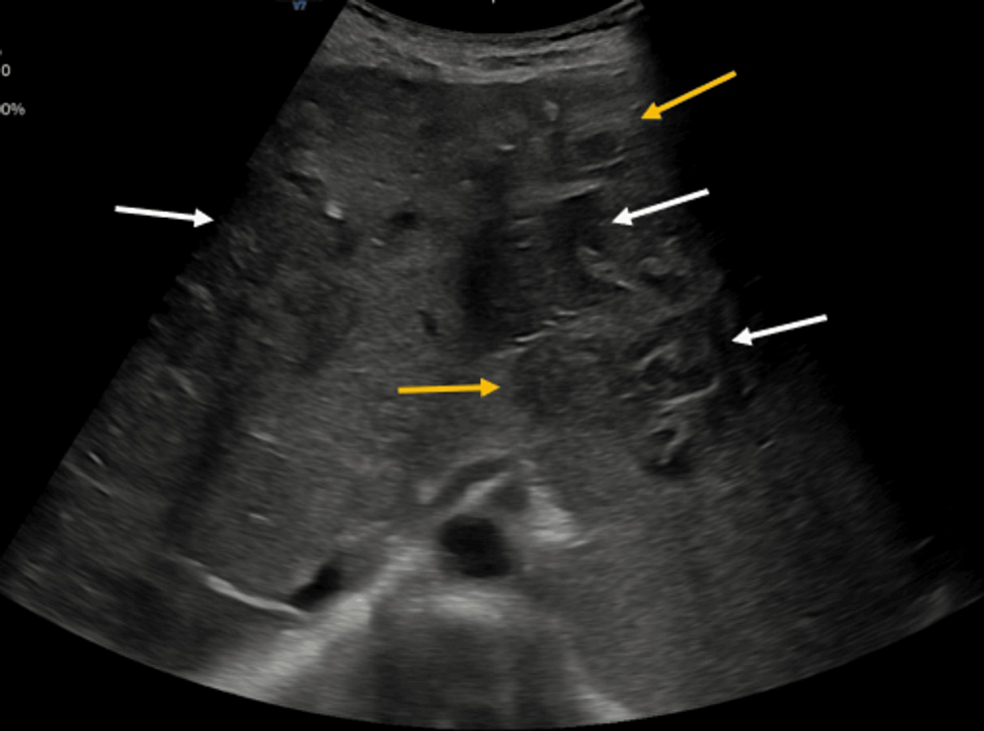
On ultrasound, multiple hypoechoic lesions are noted scattered throughout the liver parenchyma (white arrows). Some lesions show a target ring appearance that is a central hypoechoic area surrounded by hyperechoic rims (yellow arrows).
